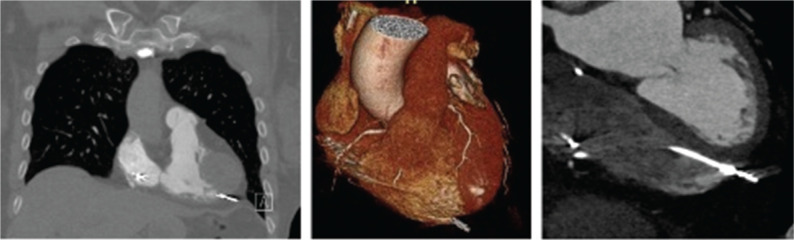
Computed tomography angiography demonstrating right ventricular lead perforation. A 3-dimensional reconstruction of the heart is shown in the center image. The tip of the lead is piercing the myocardium and extending outside the heart border.
